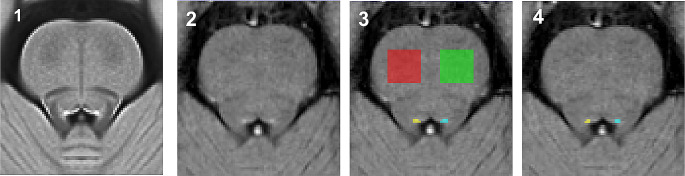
Template-based method for LC-MRI parameters estimation. LC images acquired in included subjects were warped together to build a brainstem template; signal intensity threshold is calculated, and LC mask extracted (1). Please note that the latter step refers to the whole cohort, published in Galgani et al. 2023. Then, the single patient LC sequence was warped into the brainstem template (2). LC mask was superimposed and LC_CR_ parameter was extrapolated (3). A subject-specific threshold was calculated to compute the LC_VOX_ parameter (4)
